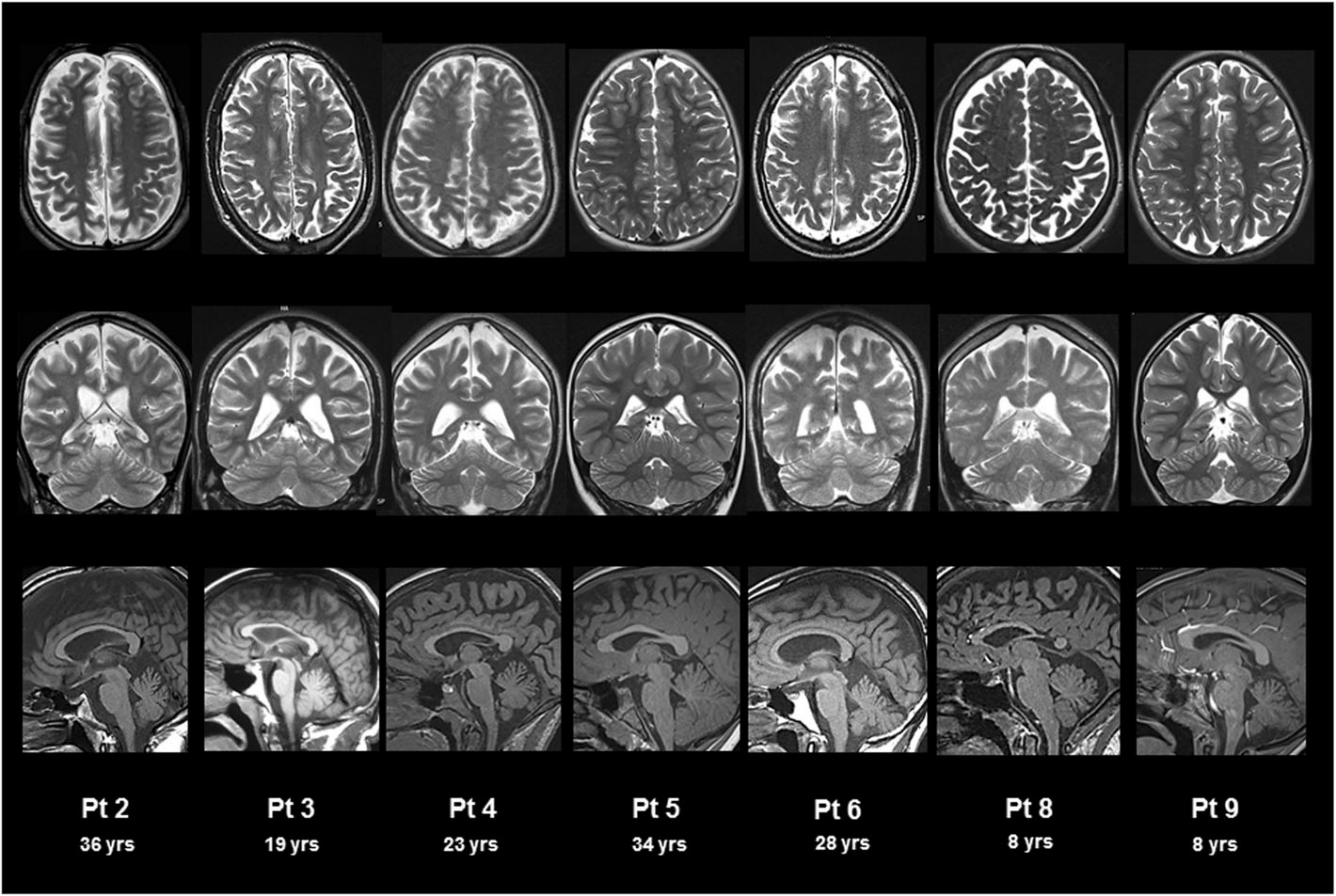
Brain MRI in patients with HHH syndrome. T2 weighted axial (upper panel), coronal (middle panel), and T1 weighted sagittal (lower panel) brain MRI. Atrophy of variable degree is detected in supra-tentorial region (moderate in patients #2, 3, 5 and 6; mild in patient # 4; absent in patients #8, and 9), corpus callosum (moderate in patients #3 and 5; mild in patients # 2, 4 and 6; absent in patients # 8 and 9) and cerebellum (mild in patients #2, 4, 5, 6 and 9, absent in patients # 3, and 8)
